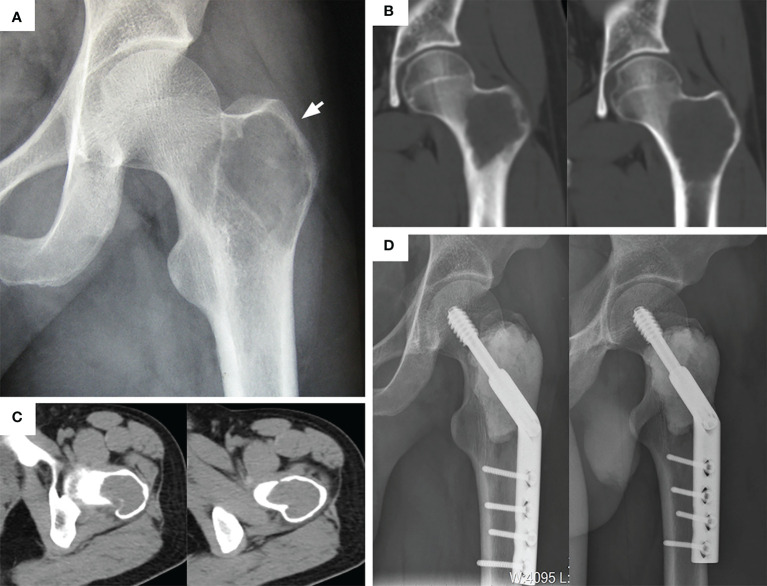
Typical preoperative and postoperative manifestations of EC for H2 type GCTB of proximal femur. (A) Apparent osteolytic lesions can be seen in the greater trochanter (arrow). (B) A coronal plane CT scan showed that the tumor invaded the femoral neck and intertrochanteric space. (C) CT transverse section showed that the bone cortex around the lesion was thin but not completely penetrated. (D) After extended curettage, allogeneic bone and bone cement filling, and DHS plate fixation. The anteroposterior and lateral radiographs was rechecked at 29 months.
